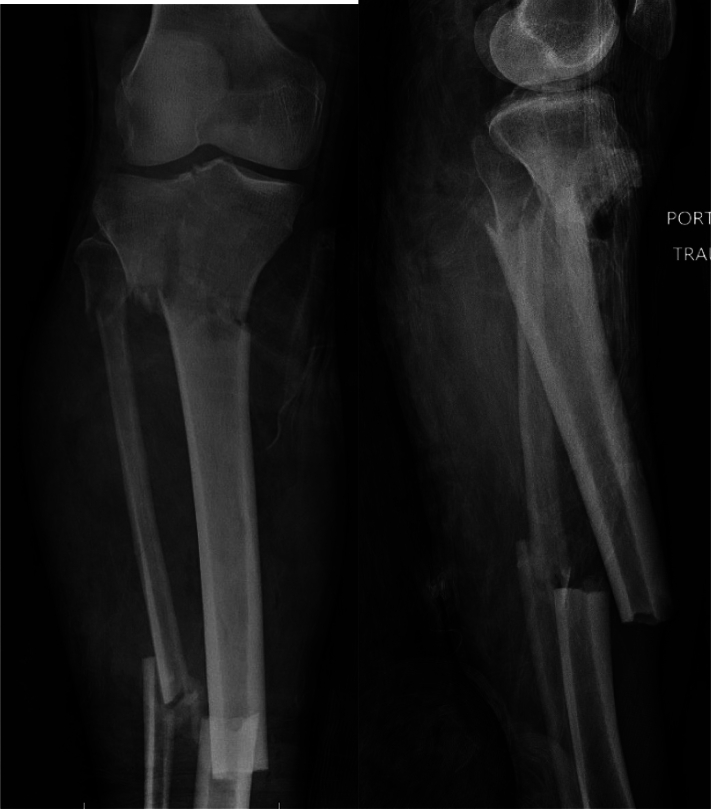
AP and lateral radiographs of a 54-year-old male (Case 13) involved in a building collapse/crush injury demonstrating 41C3/42A3 tibia fracture with multi-articular split-depression proximal tibia fracture with metaphyseal comminution, and concomitant ipsilateral transverse tibial shaft fracture.
